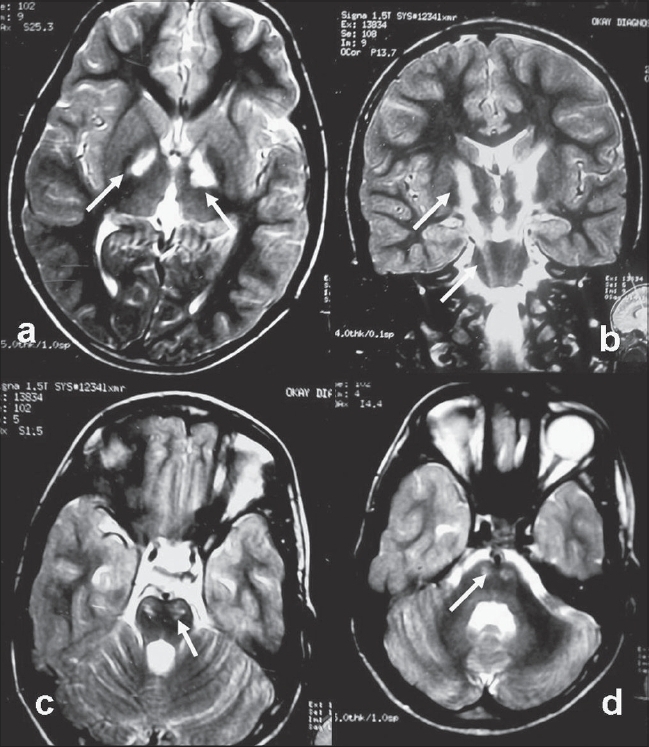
MRI Brain T2W images showing symmetrical hyperintensity (indicated by arrows) along the bilateral descending tracts in the internal capsule (axial image (a) and coronal image (b) and through the brain stem in the pons (c) and medulla (d), with associated brain stem atrophy
